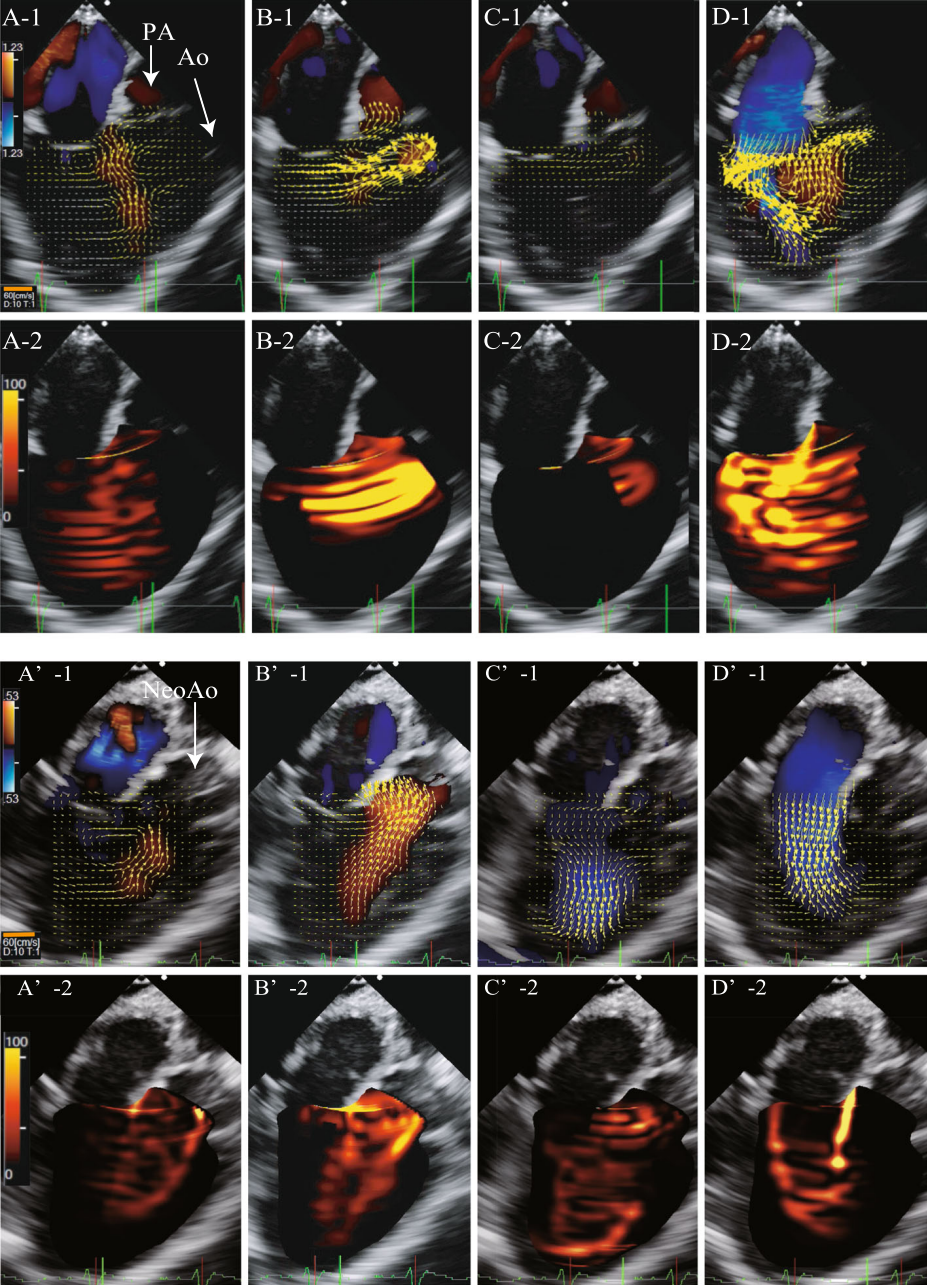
Vector flow mapping by transesophageal echocardiography. Vector flow mapping (VFM) of the mid-esophageal long-axis view before (A–D) and after (A'–D') cardiopulmonary bypass. Brightness indicates high energy loss. After the surgery, EL decreased dramatically, especially in the diastolic phase. A,A’:isovolumic contraction phase. B,B’:systolic phase. C,C’:isovolumic relaxation phase. D,D’:Diastolic phase. -1: vector flow mapping image, orange line is scale bar of the vector. -2: Energy loss image. Abbreviations: Ao, aorta; EL, energy loss; Neo Ao, neo aorta; PA, pulmonary artery
